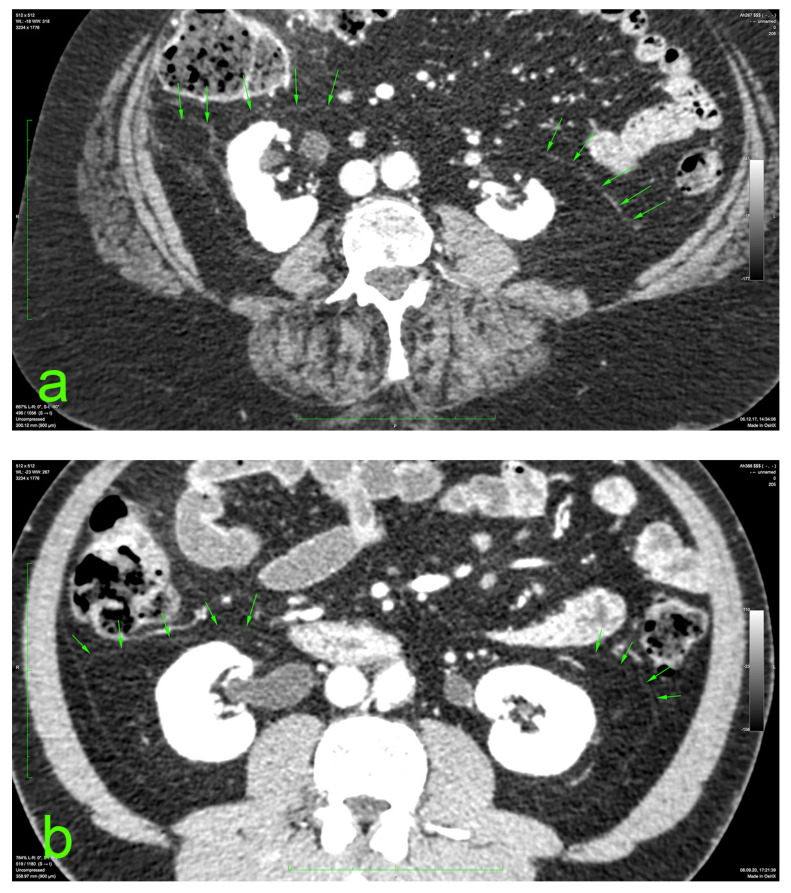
Prerenal fasciae (green arrows) on injected CT: (a) 70-year-old female patient with a BMI of 32.9; (b) 50-year-old male patient with a BMI of 30.1.
